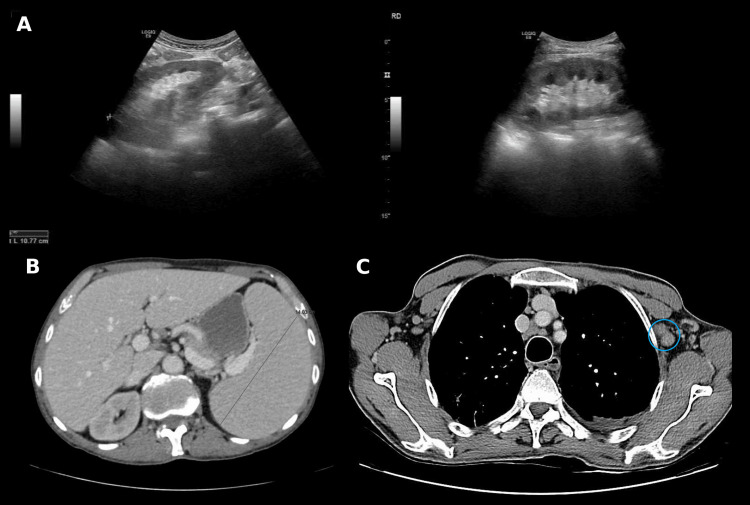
Radiological imaging (A) Renal ultrasound showing the left kidney (left panel) and right kidney (right panel), both normal-sized with diffuse cortical hyperechogenicity. (B) Axial CT of the abdomen demonstrating mild splenomegaly (spleen measuring 14.03 cm). (C) Axial CT of the thorax demonstrating an enlarged left axillary lymph node (14 × 18 mm; blue circle).
